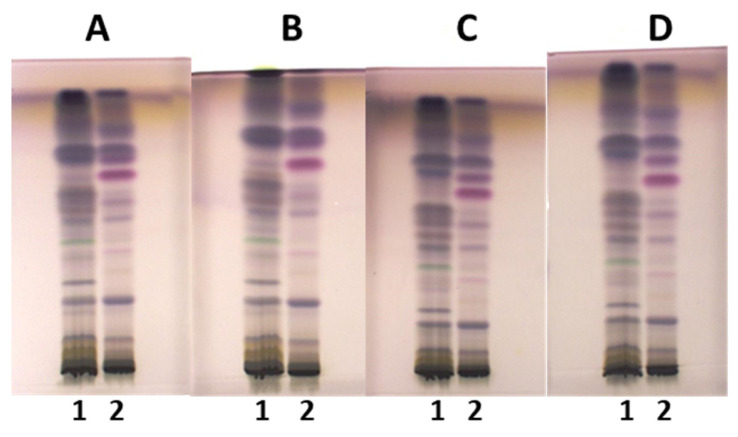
The influence of the developing solvents, toluene-ethyl acetate-methanol (5:4:1, *V/V/V*) (A,B) and toluene-isopropyl acetate-methanol (5:4:1, *V/V/V*) (C,D), and the developing distance ((A,C): 8 cm; (B,D): 9 cm) on the separation of the methanolic extracts (ultrasound-assisted extraction; 10 µL, 200 mg/mL) on the HPTLC silica gel F_254_ plates developed in a saturated (10 min) twin-trough chamber. Documentation was performed under white light after derivatization with anisaldehyde reagent. Track 1: young shoots SI2; track 2: bark from 1–2-year-old branches SI3.
